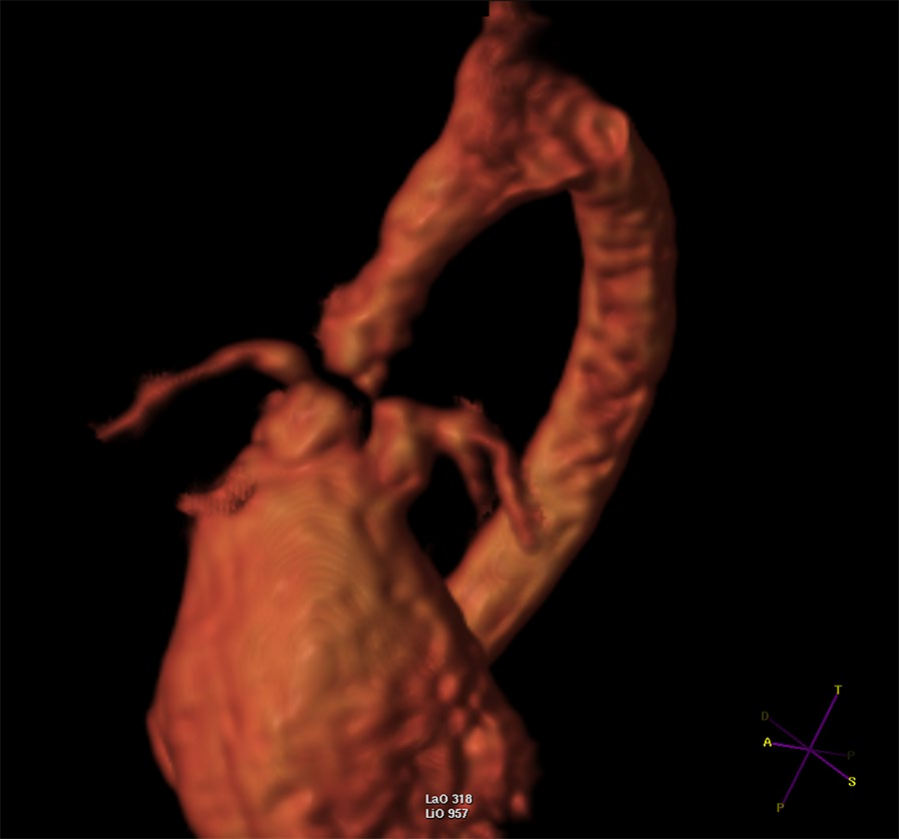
Heart MRI scan showing multiple stenotic and aneurysmatic tracts along the whole coronary artery tree
